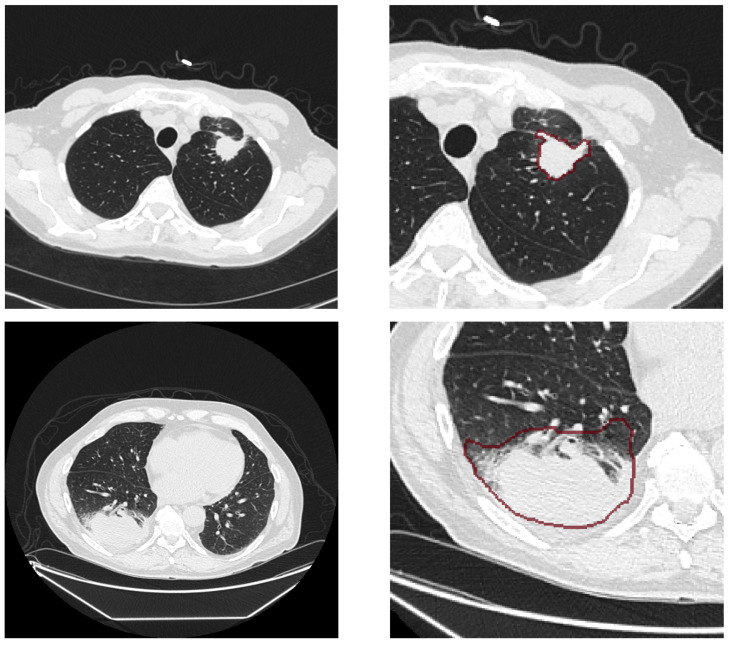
A visualization of CT images (left) and their corresponding segmentation (right, indicated by red line) from the TCIA dataset, showcasing the differences in segmentation precision and the inclusion of air or lung parenchyma in R01-075 (top) and R01-069 (bottom).
